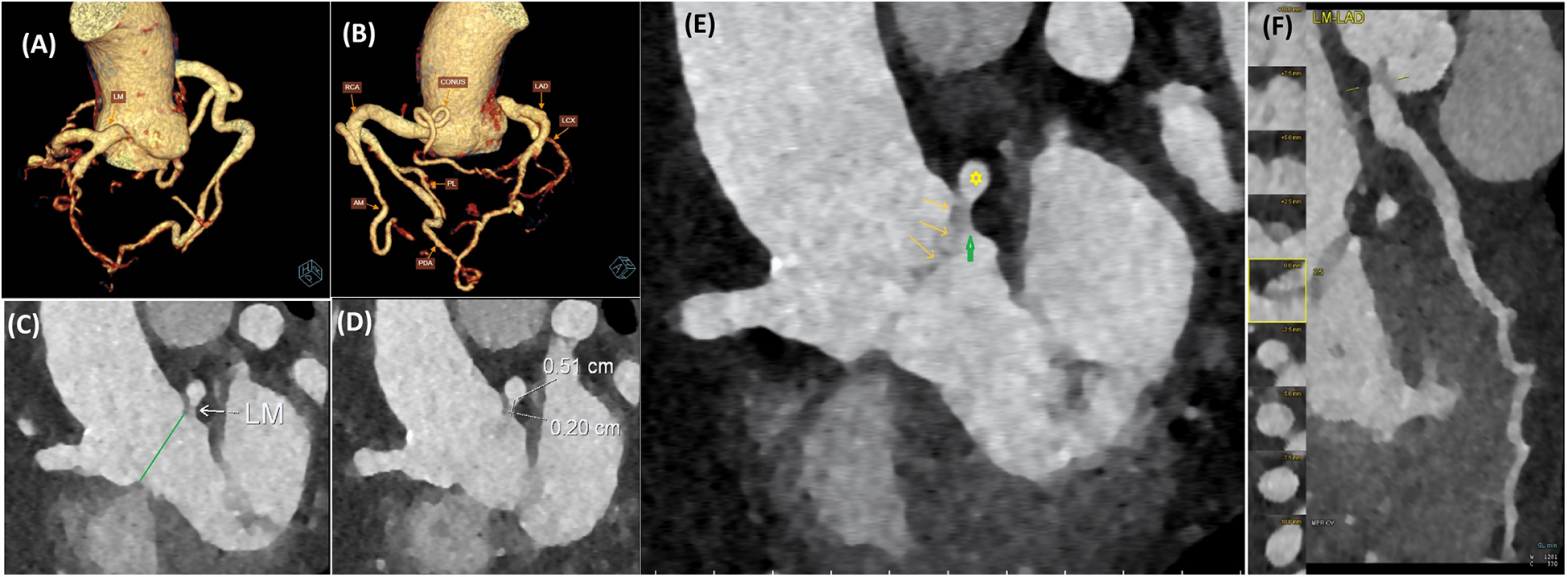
Coronary computed tomography angiography shows the origin of the LMCA below the aortic valve (originating from the LVOT). (A) 3D CT reconstruction shows the LMCA origin below the aortic annular plane at the commissure between the left coronary cusp and the non-coronary cusp. (B) 3D CT reconstruction shows a dilated RCA and collateral circulation from the RCA to the left coronary system. (C) The LMCA originated below the aortic annular plane (green line). (D) Ostial narrowing and the intramural segment of LMCA measurements. (E) Relationship of LMCA trunk (yellow asterisk) and hypoplastic ostium (green arrow) to aortic valve annulus and aortic valve leaflets (yellow arrows). (F) LMCA-LAD artery course reconstruction. LM, left main coronary artery; LAD, left anterior descending artery; LCX, left circumflex artery; RCA, right coronary artery; CONUS, conus branch of the right coronary artery; AM, the acute marginal artery; PDA, posterior descending artery; PL, posterolateral branch artery.
